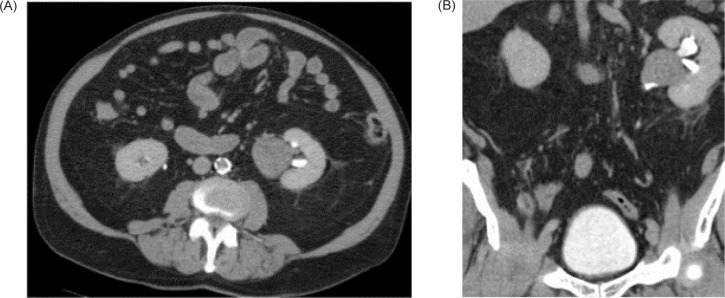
Left renal pelvis mass on delayed phase of CT Urogram. (A) Axial (B) Coronal
